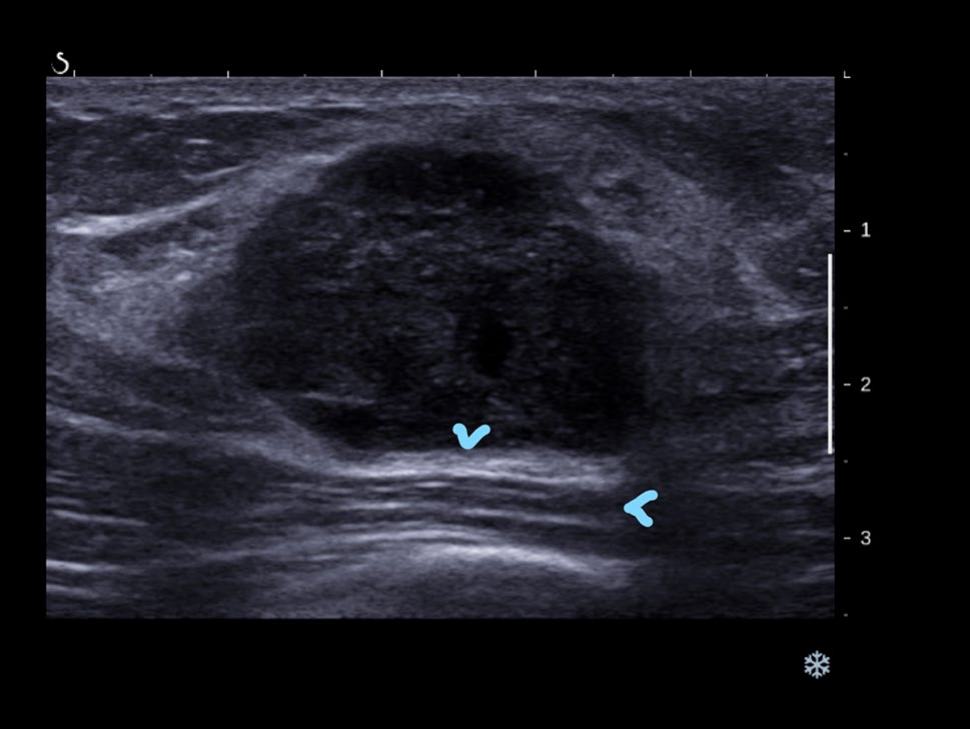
Ultrasound image of a triple negative breast cancer showing posterior echo enhancement as shown by the arrow heads
